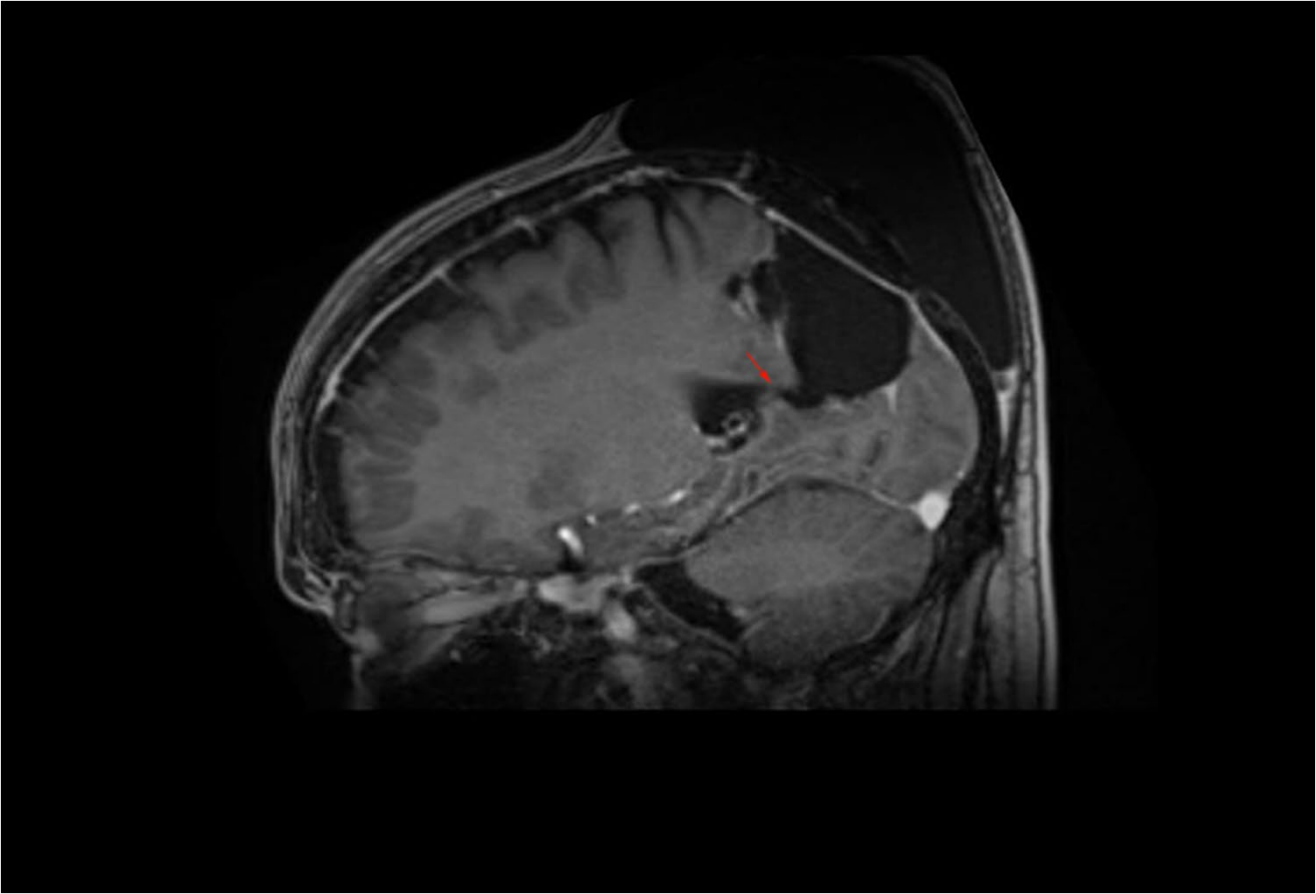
Preoperative sagittal T1-weighted MRI showing the tumor bed cyst and its communication with the epicranial CSF collection. Red arrow points to the location of the slit valve between the posterior horn of the lateral ventricle and the tumor bed
